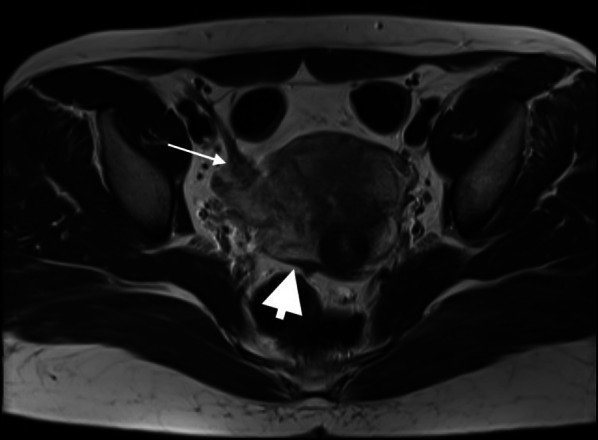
T2 weighted axial MRI image showed involvement of right uterosacral (thick arrowhead) and round ligaments (thin arrow) by endometriosis showing as nodularities, irregularities, and thickening
